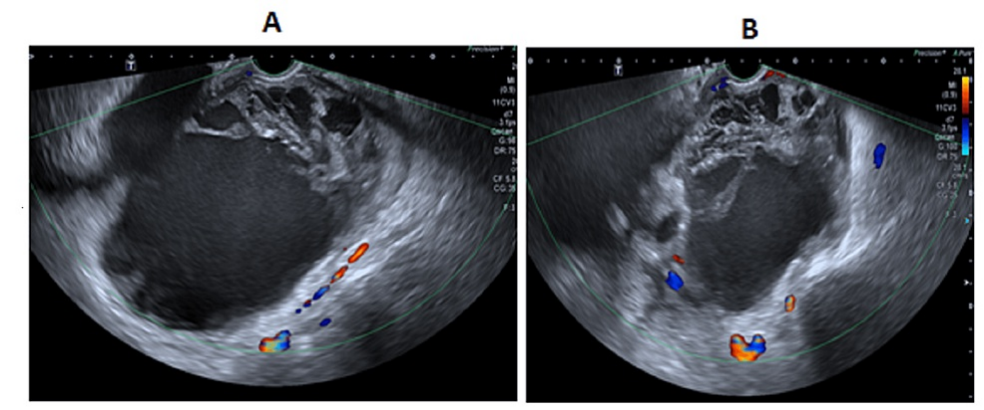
Ultrasound images (second ED visit) A follow-up pelvic scan showing a pelvic collection of 15.3 × 11.7 × 11.9 cm, 1122.3 cc in volume (previously 332 mL). ED, emergency department
